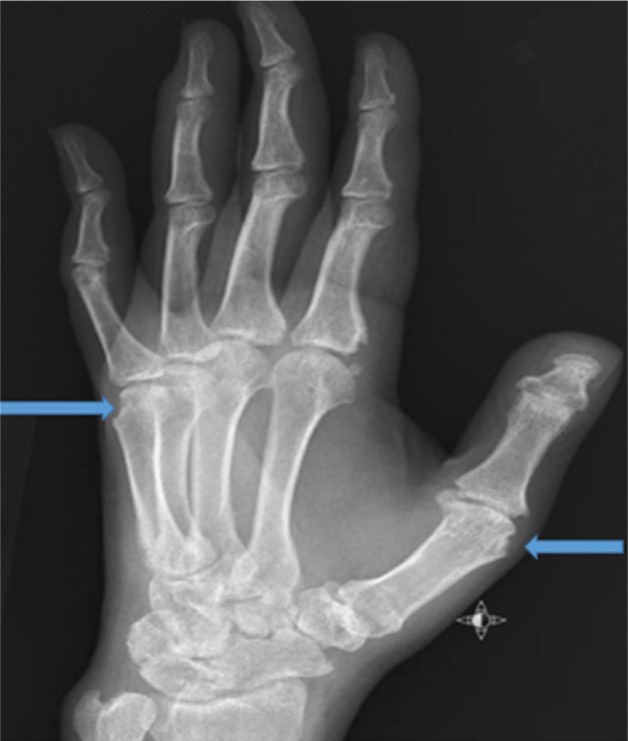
Hand X-ray showing erosions.
